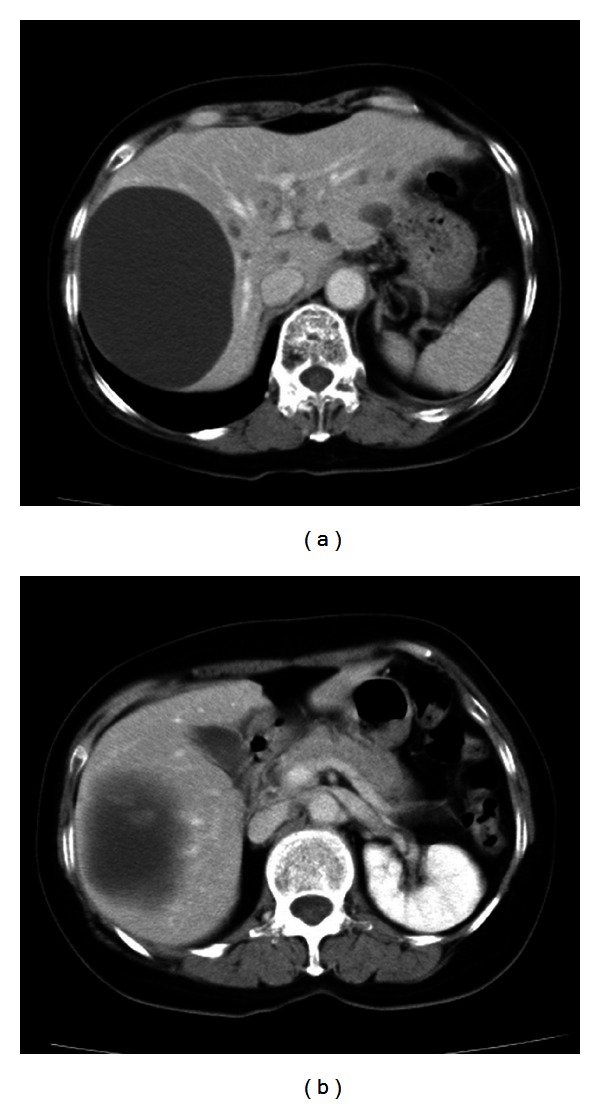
Abdominal contrast-enhanced computed tomography performed in September 2011 shows multiple cysts in both hepatic lobes. Nodular structures can be observed on the caudal side of a giant cyst.
